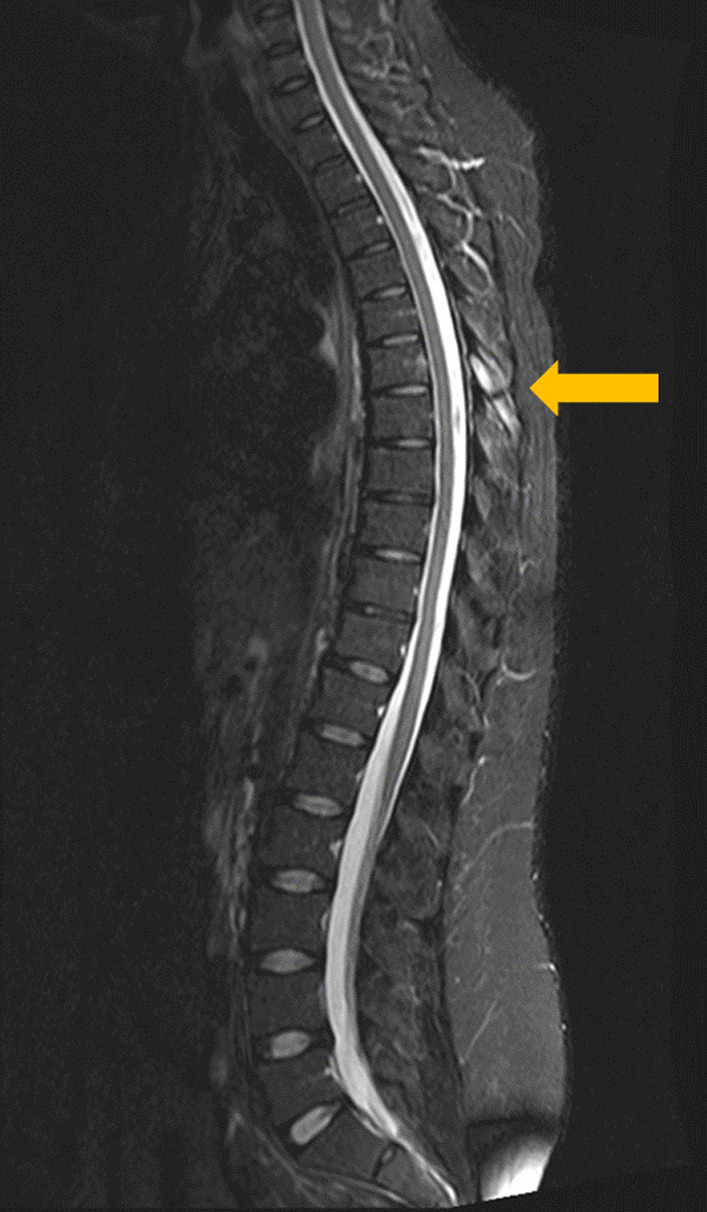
Short tau inversion recovery sequence magnetic resonance imaging sagittal view showing an isolated T6 nonunion (gold arrow) 2 years after the fracture
